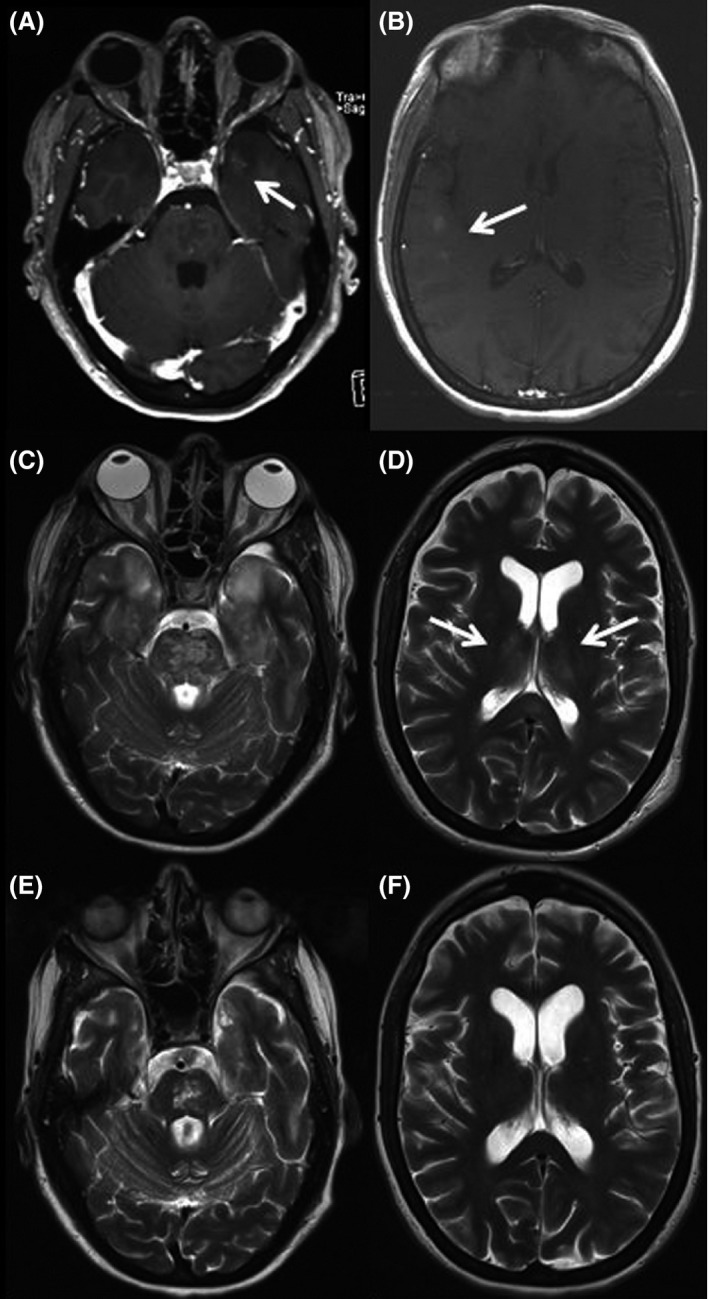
Serial contrast‐enhanced (A + B) and T2‐weighted (C‐F) MRI.
